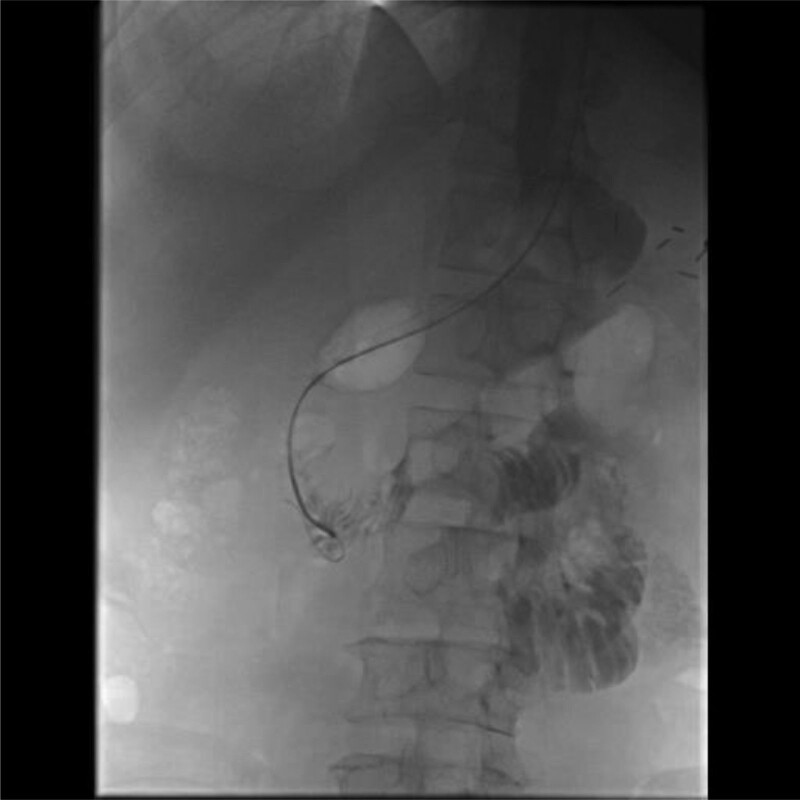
Upper gastrointestinal radiography showed duodenal end leakage.
